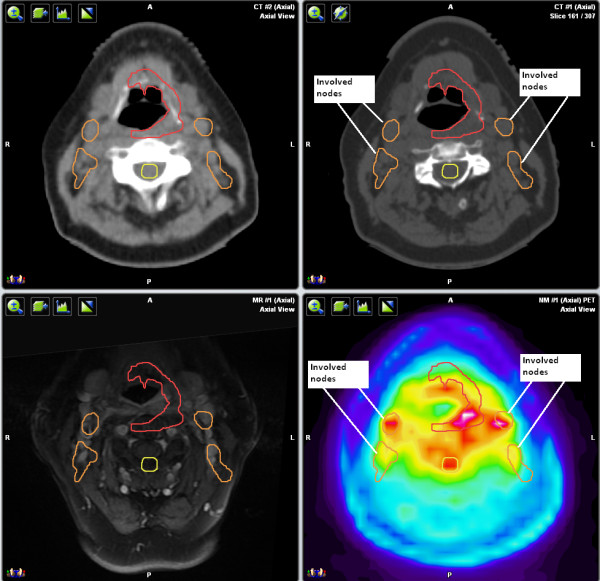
CT, MRI, and PET axial views. Involvement of the bilateral subcentimeter regional lymph nodes was appreciated on PET, but not on CT or MRI.
